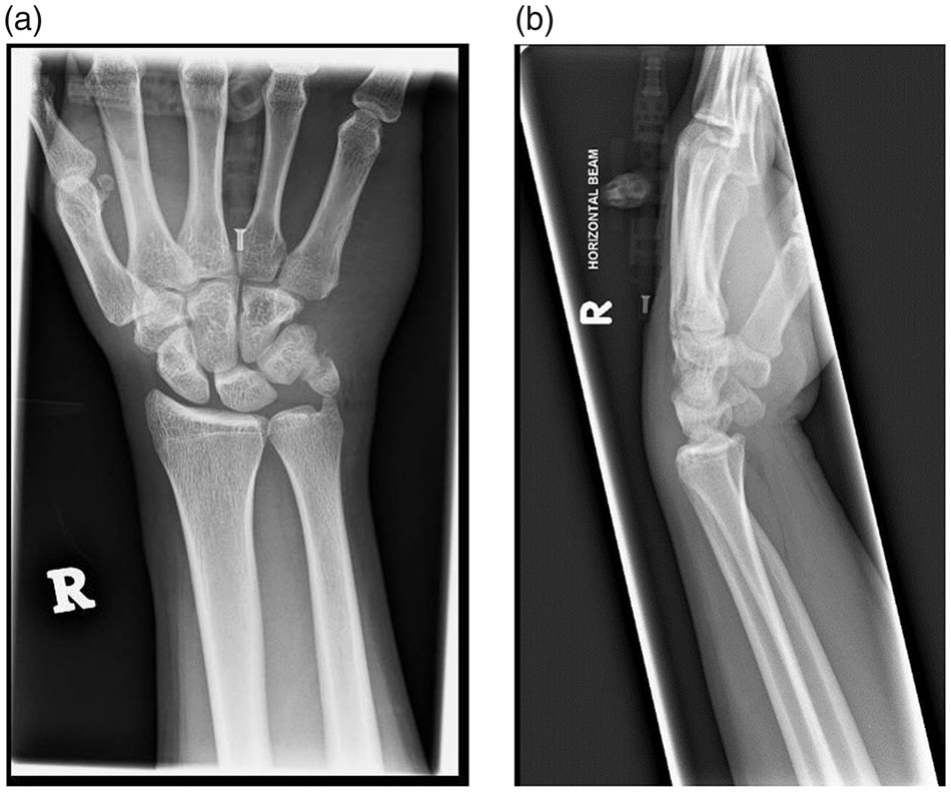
(a) X-ray (anteroposterior view) of the right hand, showing the displaced pisiform fracture. (b) X-ray (lateral view) of the right hand, showing the displaced pisiform fracture.
